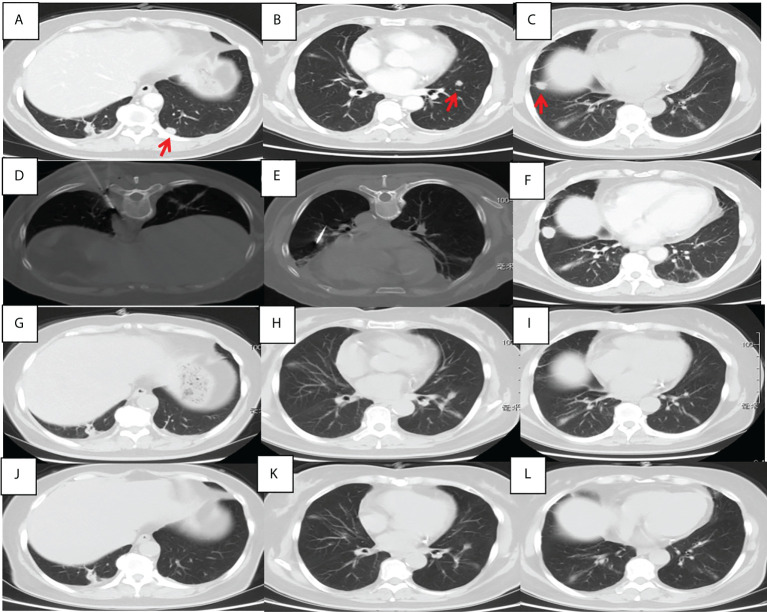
Chest CT findings of a 56-year-old female pulmonary adenocarcinoma patient with an unknown PD-L1 TPS who underwent MWA and camrelizumab monotherapy. (A–C) Baseline chest CT showing two metastases in the left lower lobe. (D, E) MWA was conducted for the tumor in the left lower lobe. (F) Two cycles of camrelizumab monotherapy were administered, after which the right metastasis enlarged. (G–I) Six months post-ablation, the left metastases achieved complete ablation and the right metastasis disappeared; complete response was achieved. (J–L) Nineteen months post-ablation, there was no indication of any pulmonary metastases. PD-L1, programmed death ligand-1; TPS, tumor proportion score; MWA, microwave ablation; CT, computed tomography.
